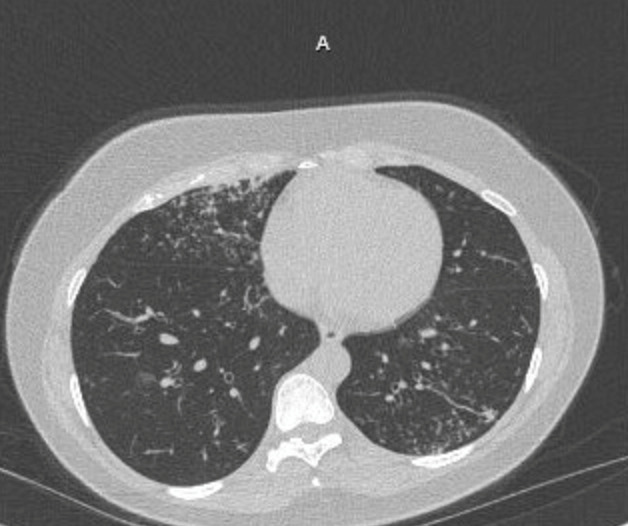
High-resolution CT scan of the chest. Extensive tree-in-bud nodules were seen throughout the bilateral lungs consistent with infectious etiology.
